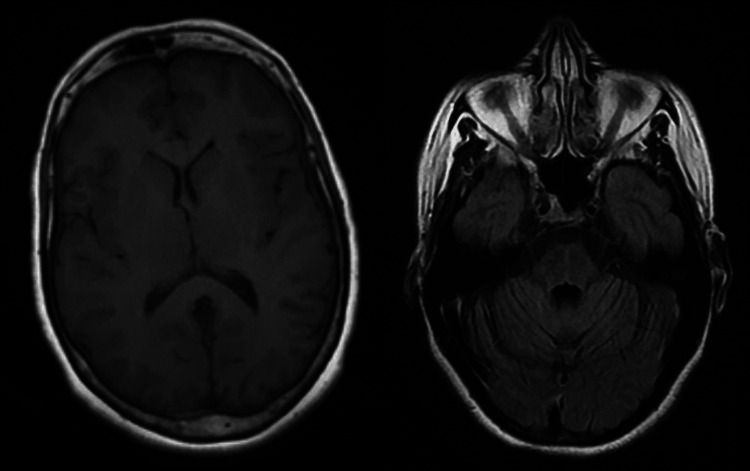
Cerebral MRI with no abnormalities
